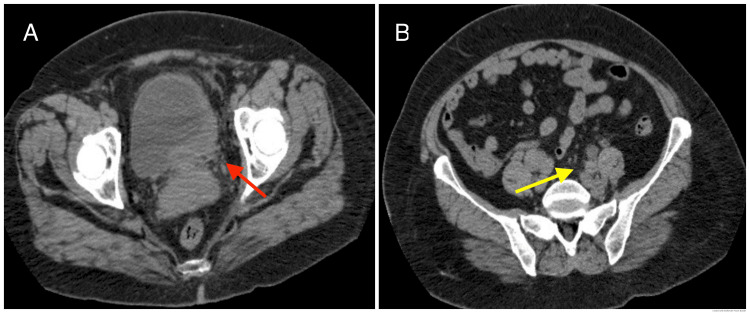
A computed tomography scan was performed for disease stratification. A: thickening of the left parasagittal aspect of the bladder (red arrow); B: common iliac suspicious adenopathies (yellow arrow).
